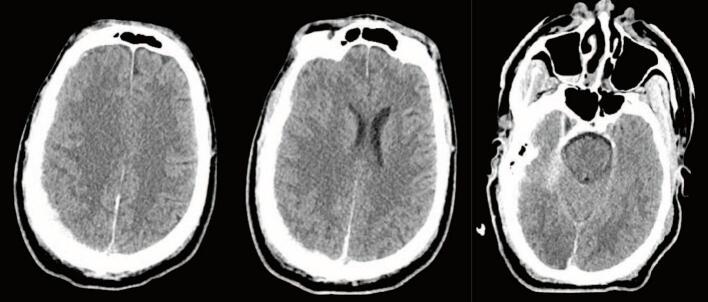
CT Head on Hospital Day 2 CT Head on HD2 showing decreased size of SDH now 6 mm and reduced midline shift of 6 mm.
